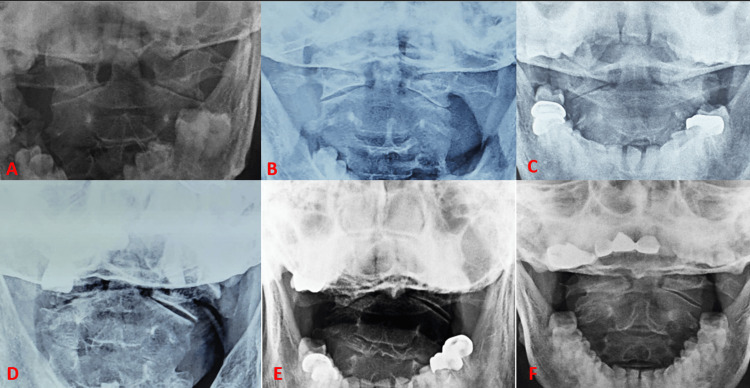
Grades of C1-C2 osteoarthritis on open-mouth radiographs. (A) Grade 1: osteophyte formation; (B) Grade 2: periarticular ossicles; (C) Grade 3: joint-space narrowing with sclerosis of subchondral bone; (D) Grade 4: subchondral cysts; (E) Grade 5: altered shape of bone ends; (F) joint destruction. C1-C2: first and second cervical vertebrae.
